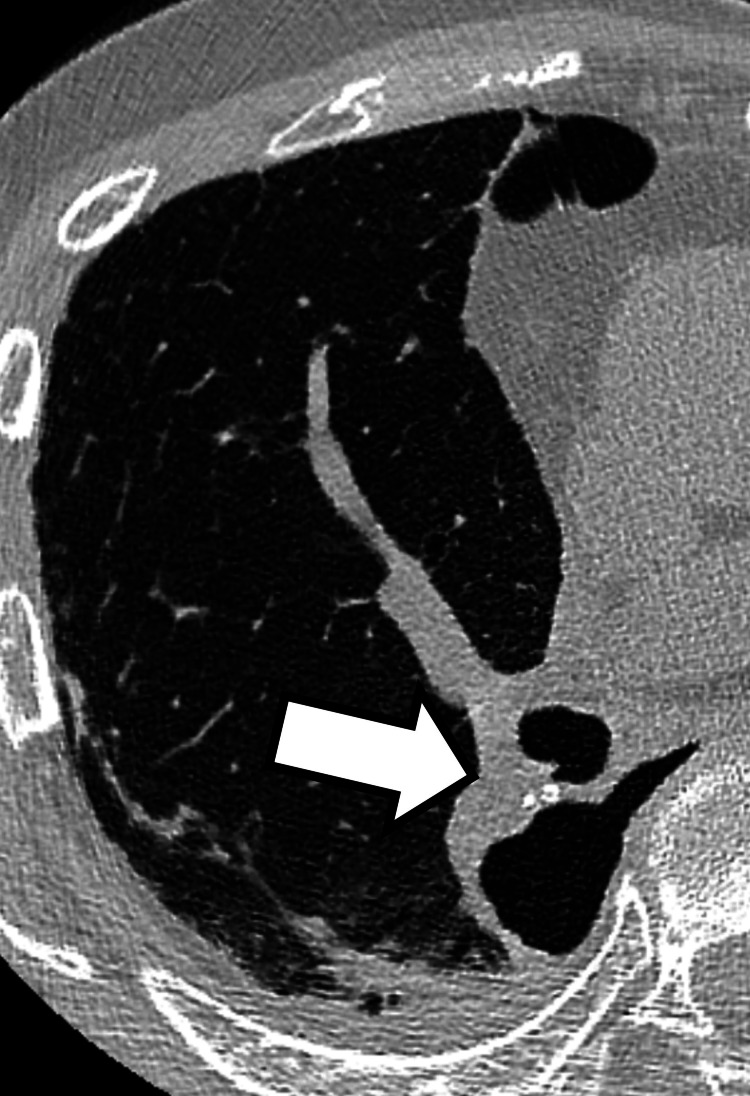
Chest CT before exploratory thoracoscopy CT image showing a pneumothorax without an obvious bronchial fistula (arrow), and no pleural effusion was observed. CT: computed tomography
